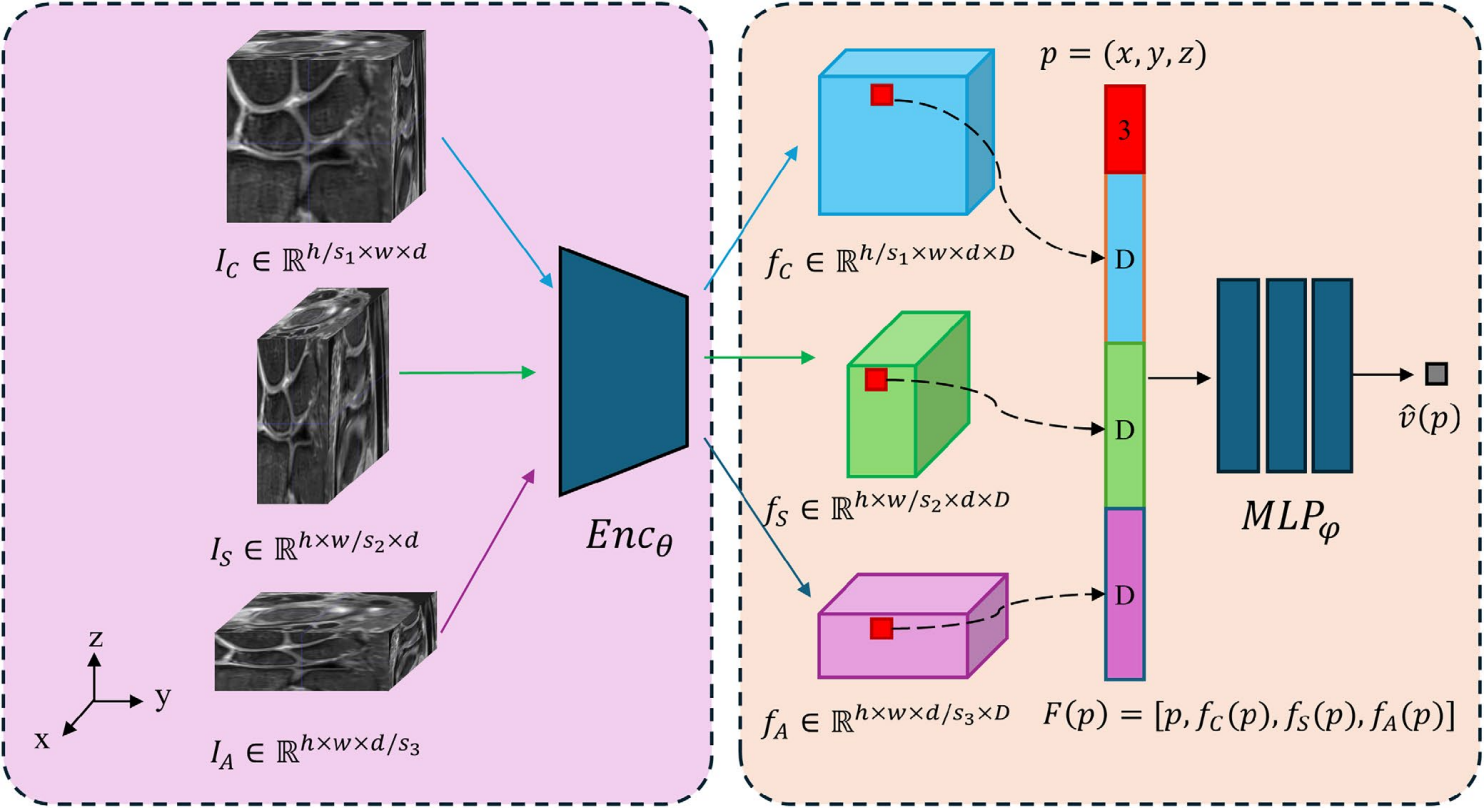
Enhancement of MRI images by implicit neural representation (INR)–based multiple‐volume isotropic super‐resolution.
